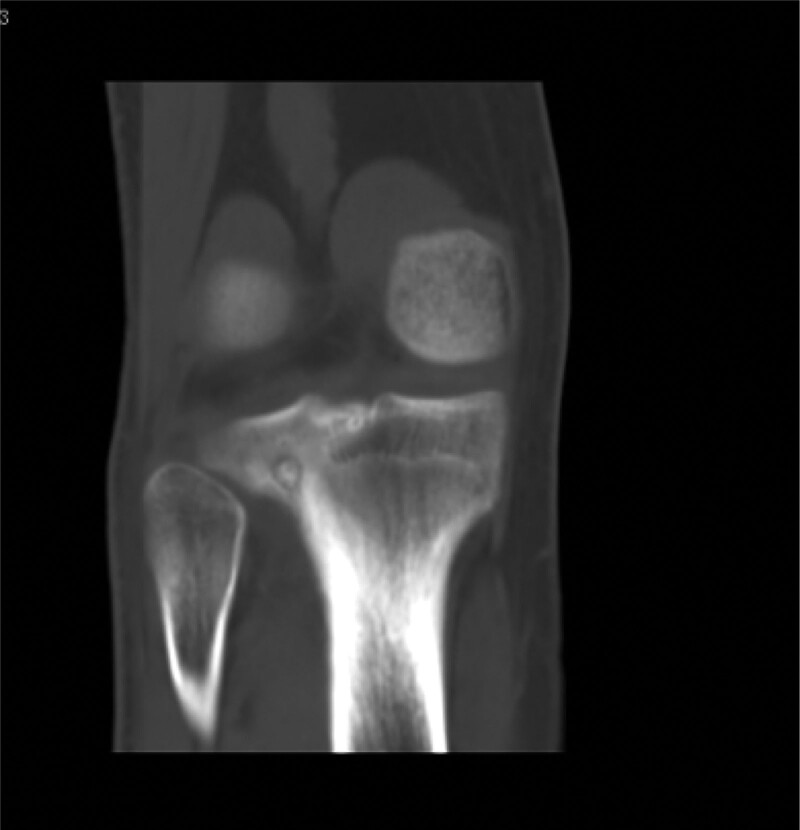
Preoperative CT image in coronal plane. CT = computed tomography.
